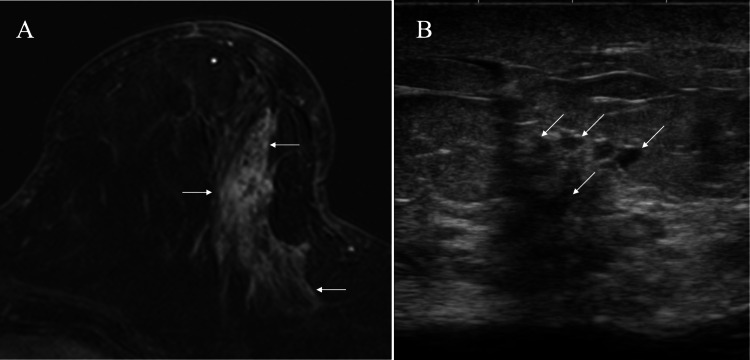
Magnetic resonance imaging (MRI) and ultrasound findings of the left breast cancer. A. MRI showed enhancement extending widely from the subnipple areas toward the left axilla (arrows); B. Ultrasound showed multiple non-mass forming lesions with internal low echoes (arrows).
